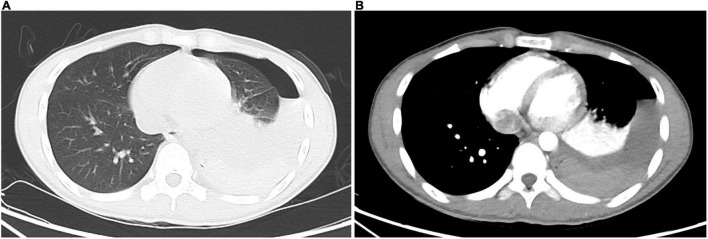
Contrast-enhanced chest computed tomography. (A) The lung window shows left hydropneumothorax; dorsal side of left lower lobe shows consolidation, which presents as atelectasis after compression. (B) The mediastinal window shows left hydropneumothorax; consolidation of the dorsal side of the left lower lobe is enhanced, which presents as atelectasis after compression; high-density lesion in the left dorsal pleural cavity has no enhancement, which is a blood clot; no blood vessels with abnormal morphology and density or contrast agent leaking into the thoracic cavity are found.
